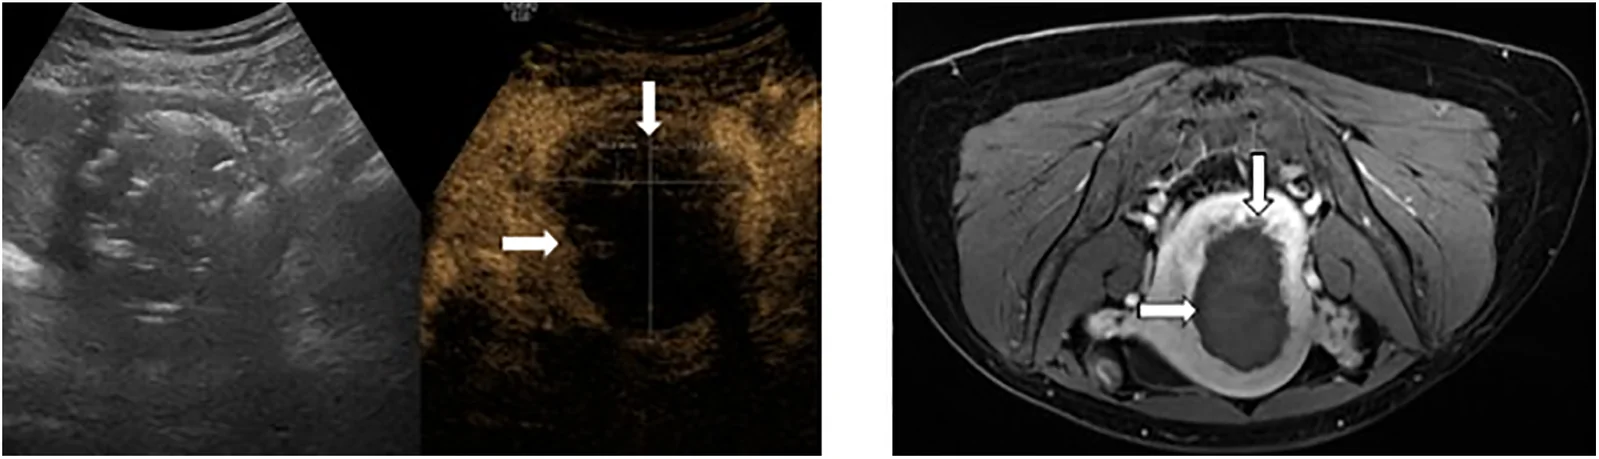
The avascular ablation zone marked by white arrows is seen as a dark area on contrast enhanced ultrasound (left) and contrast enhanced magnetic resonance imaging (right). For the ultrasound scan a transabdominal probe is used in the axial plane.
